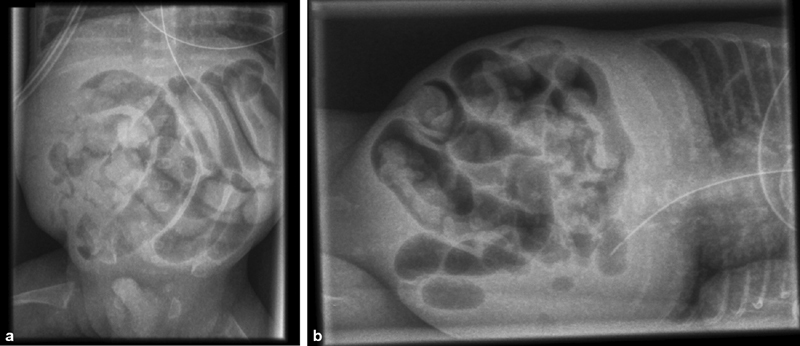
X-rays on day 14 of life in supine (
